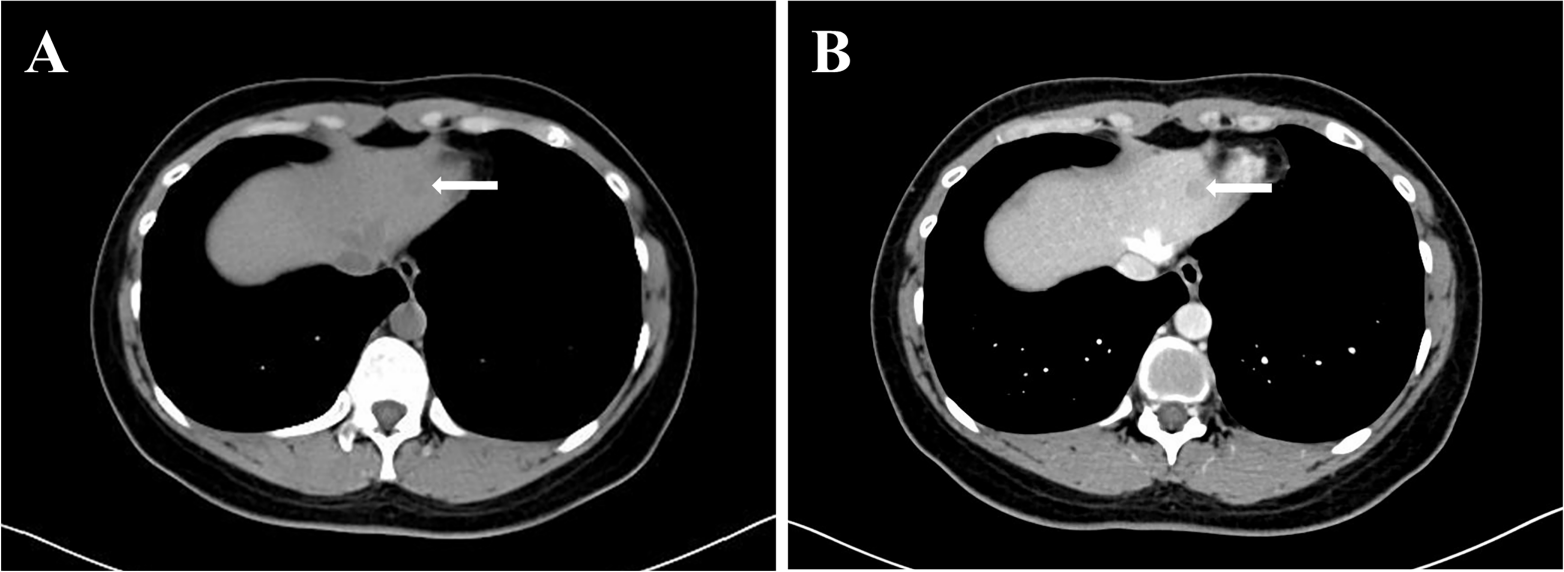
The lesion in the left lobe of liver was detected on CT.
